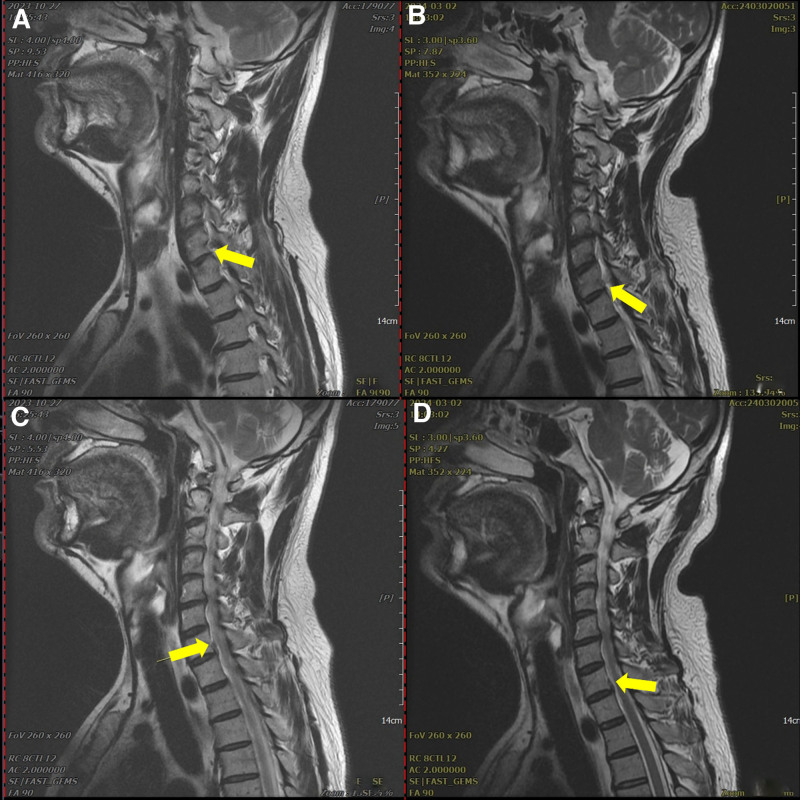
Follow-up of C-spine magnetic resonance imaging on October 27, 2023 (A, B) and March 2, 2024 (C, D).
